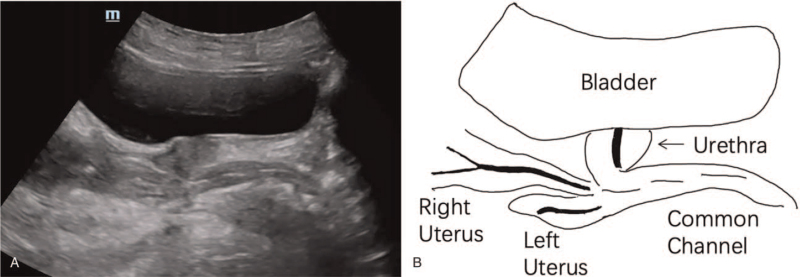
Two-dimensional transabdominal ultrasonography showing 2 vaginas and urethra.
